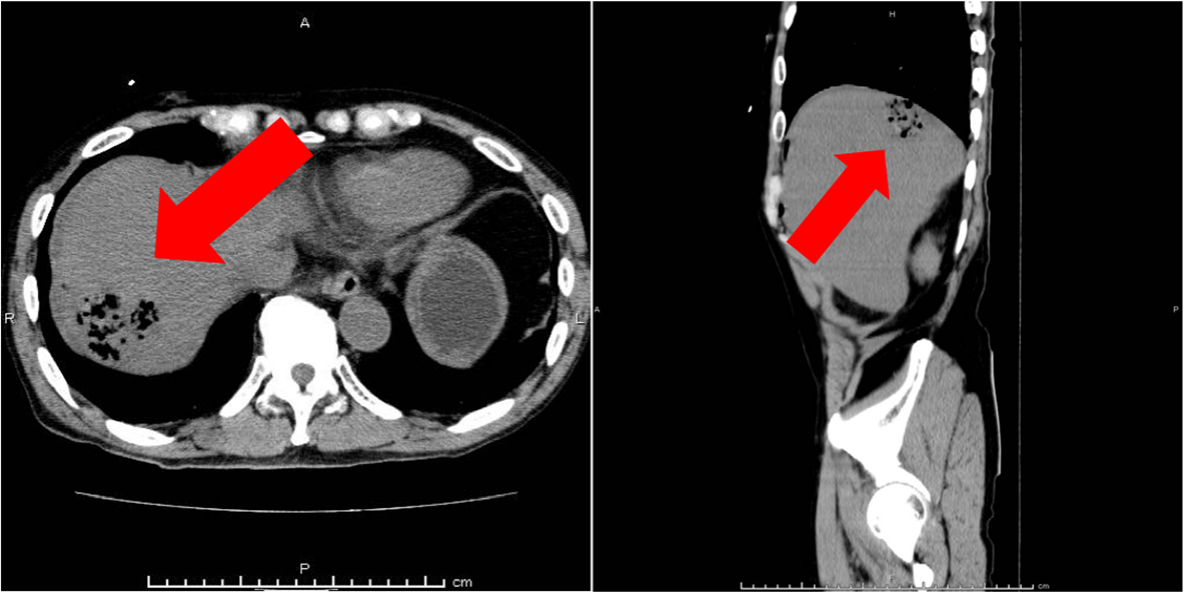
Computed tomography shows abscesses with gas in the right posterior lobes of the liver (red arrow)
